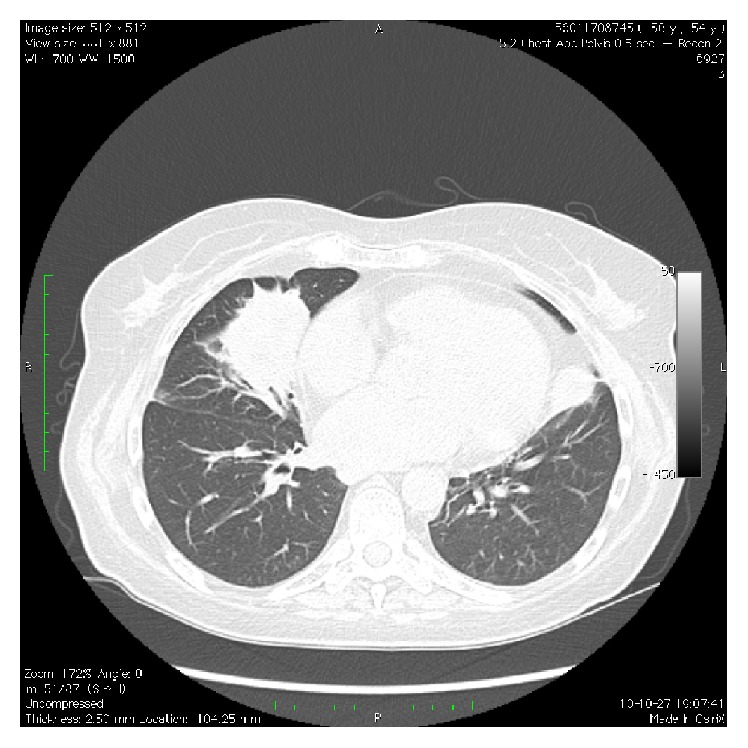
CT of the chest shows two metastatic focuses in both lungs and mediastinal nodules.
